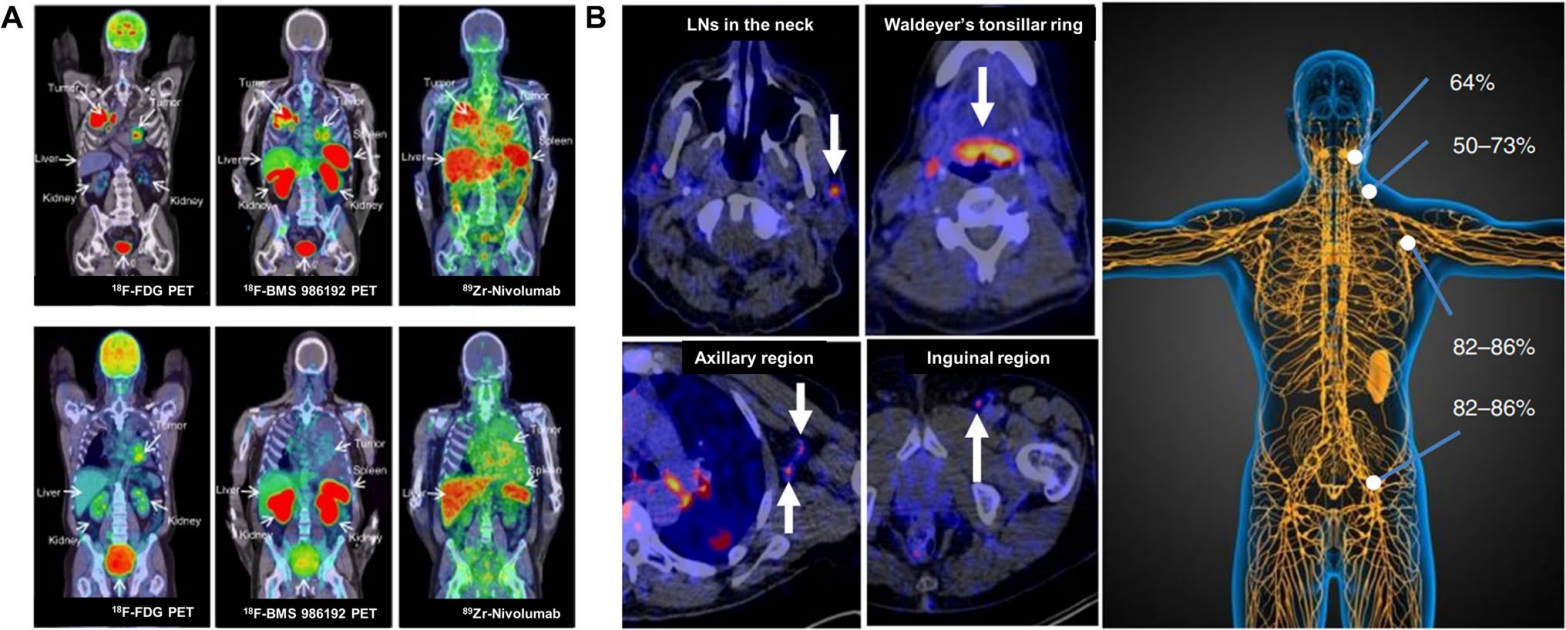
Whole-body PD(L)1 PET-imaging for the assessment of ICI therapy response. A Representative images of two patients detected by ^18^F-FDG PET, ^18^F-BMS-986192 PET, and ^89^Zr-Nivolumab PET to demonstrate heterogeneous tracer uptake. B Percentage of patients with ^89^Zr-atezolizumab uptake in healthy lymphoid tissue (right) and examples of ^89^Zr-atezolizumab uptake in the different regions (left). (The researches were originally published in Nat Commun. 2018 Nov 7;9(1):4664 [73] and Nat Med. 2018 Dec;24(12):1852-1858 [74])
